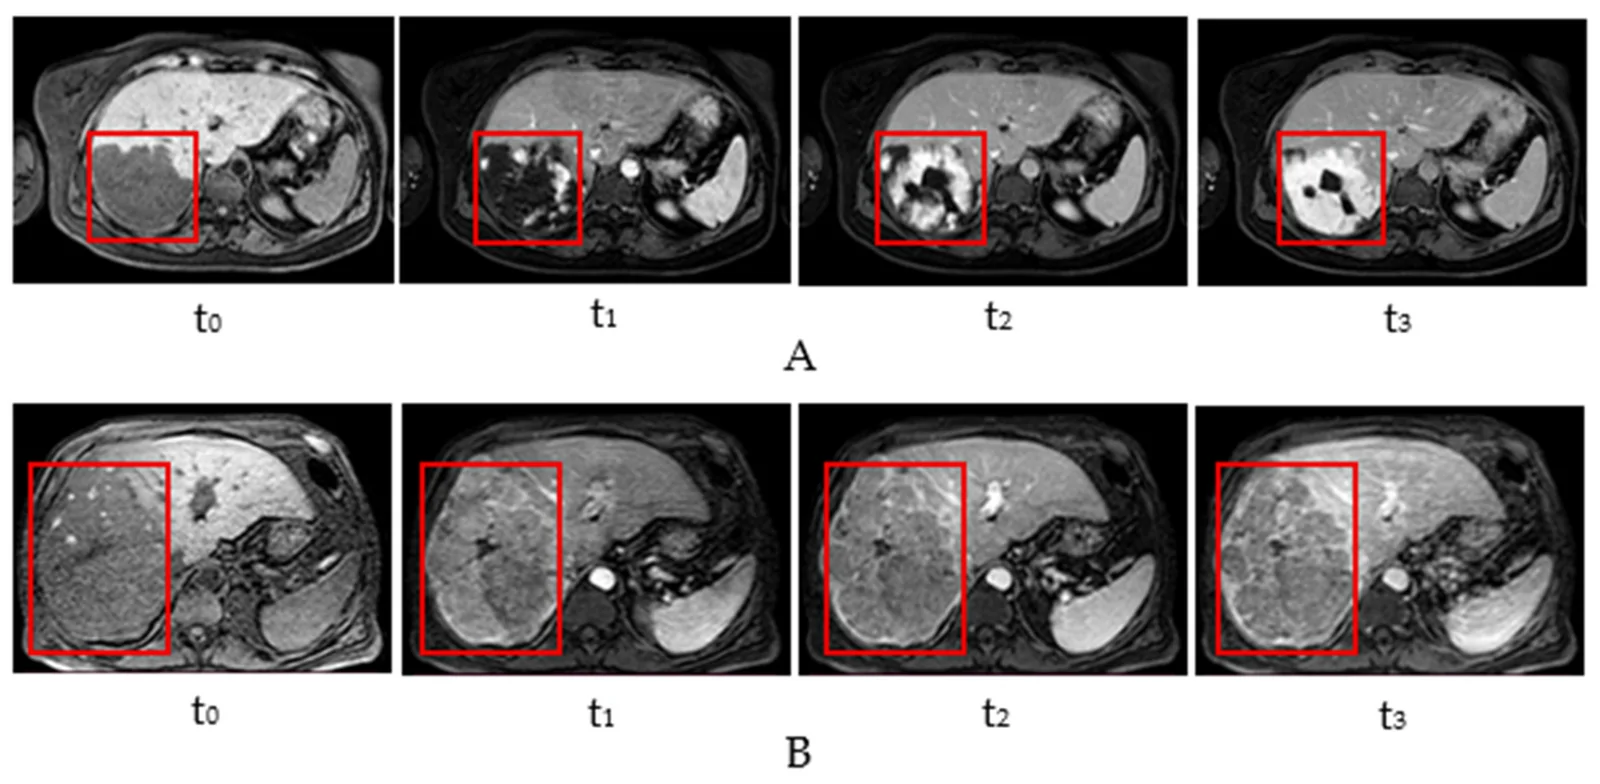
DCE-MRI scans of two patients with FLL inside the red rectangles. (A) Patient with benign FLL lesion, and (B) Patients with malignant FLL lesions.
